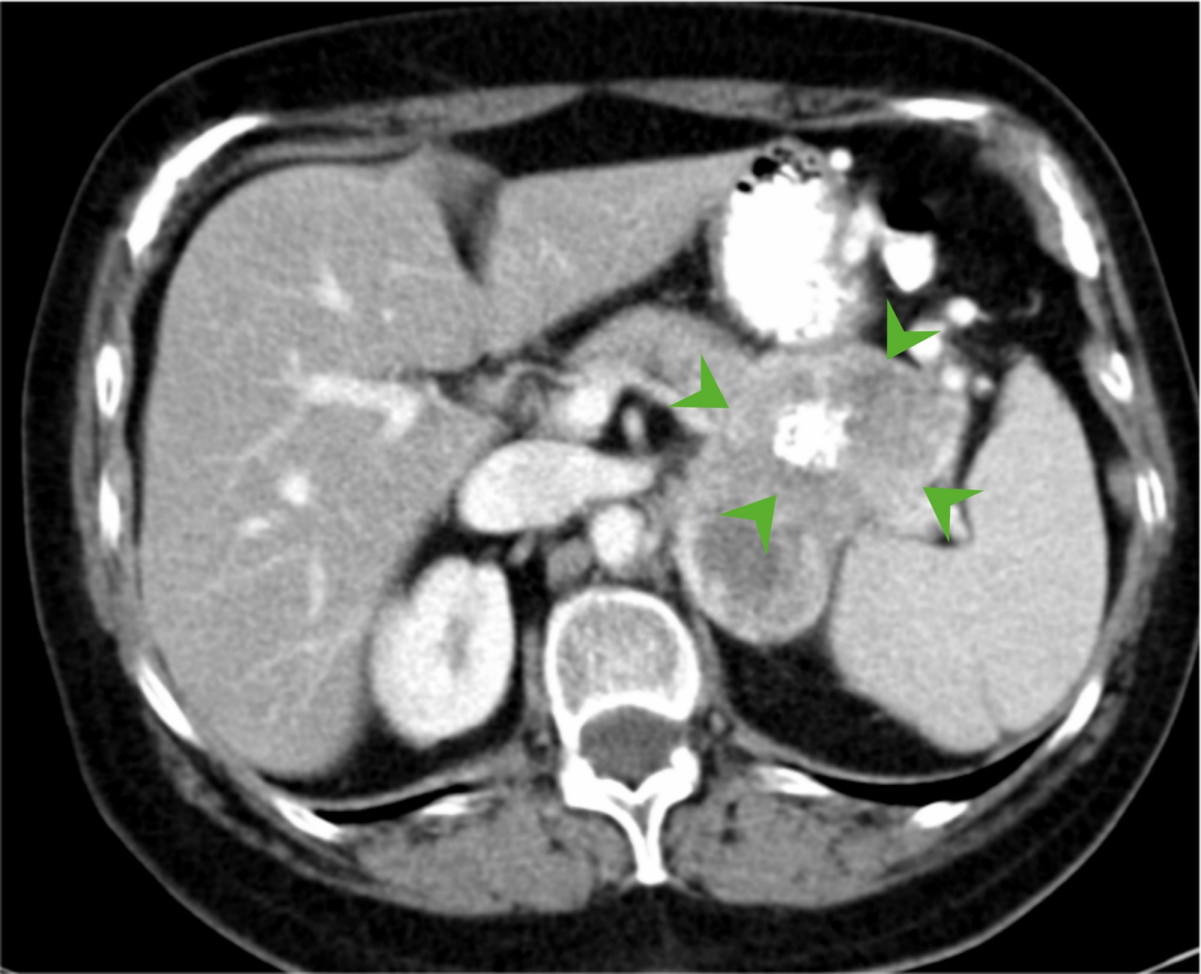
Axial contrast enhanced CT scan 11 months later. The mass has decreased in size (arrowheads).
